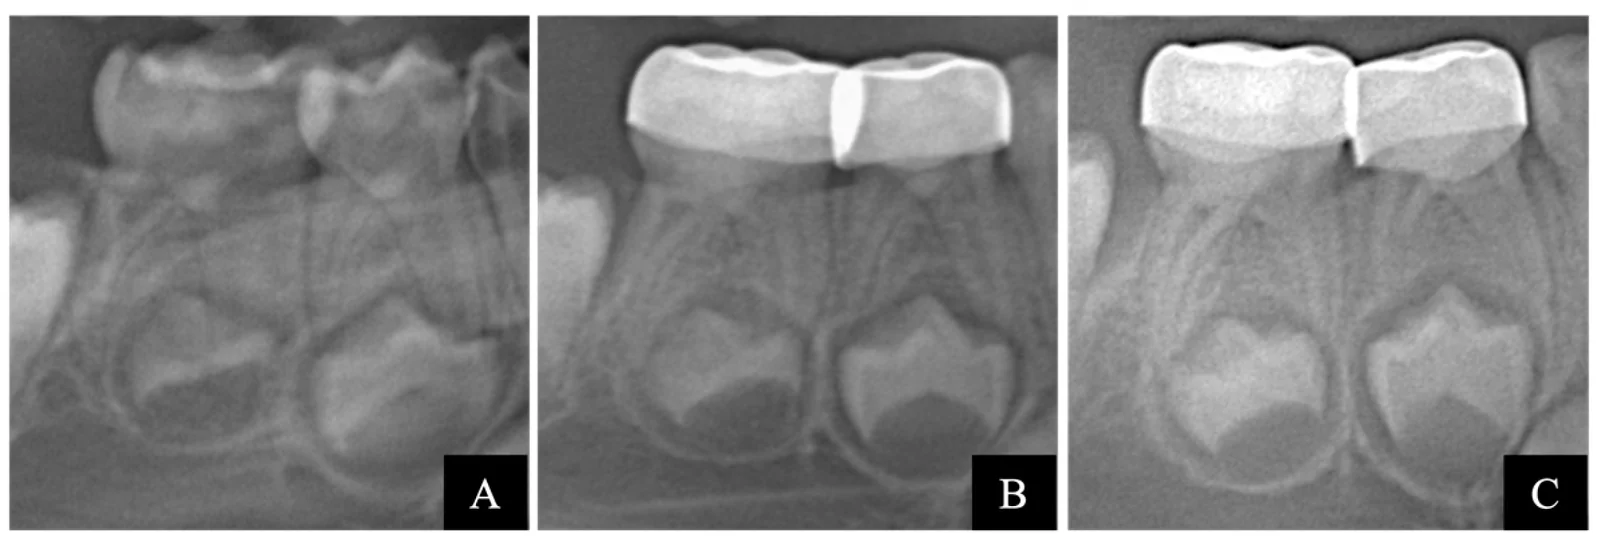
Radiographic evaluation of teeth 84 and 85 in Patient 1. (A) Preoperative radiograph; (B) 6-month post-treatment radiograph; (C) final follow-up radiograph.
